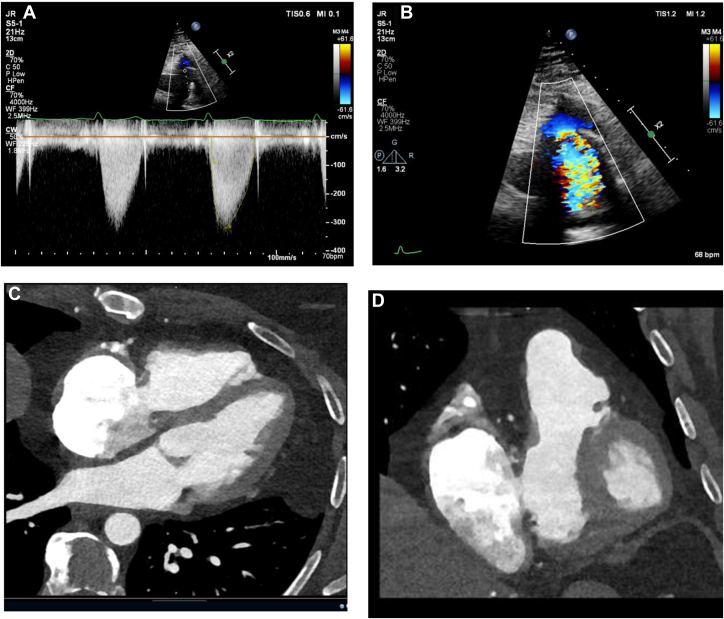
Repeat Surgical Workup (A) TTE of transvalvular PV flow indicating moderate pulmonary stenosis (PA mean PG 25.1 mm Hg, max PG 41.8 mm Hg). (B) TTE color flow Doppler demonstrating flow acceleration across PV. (C and D) Gated CT scan demonstrating likely significant thrombus burden across tricuspid valve. CT = computed tomography; PA = pulmonary artery; PG = pressure gradient; PV = pulmonary valve; TTE = transthoracic echocardiography.
